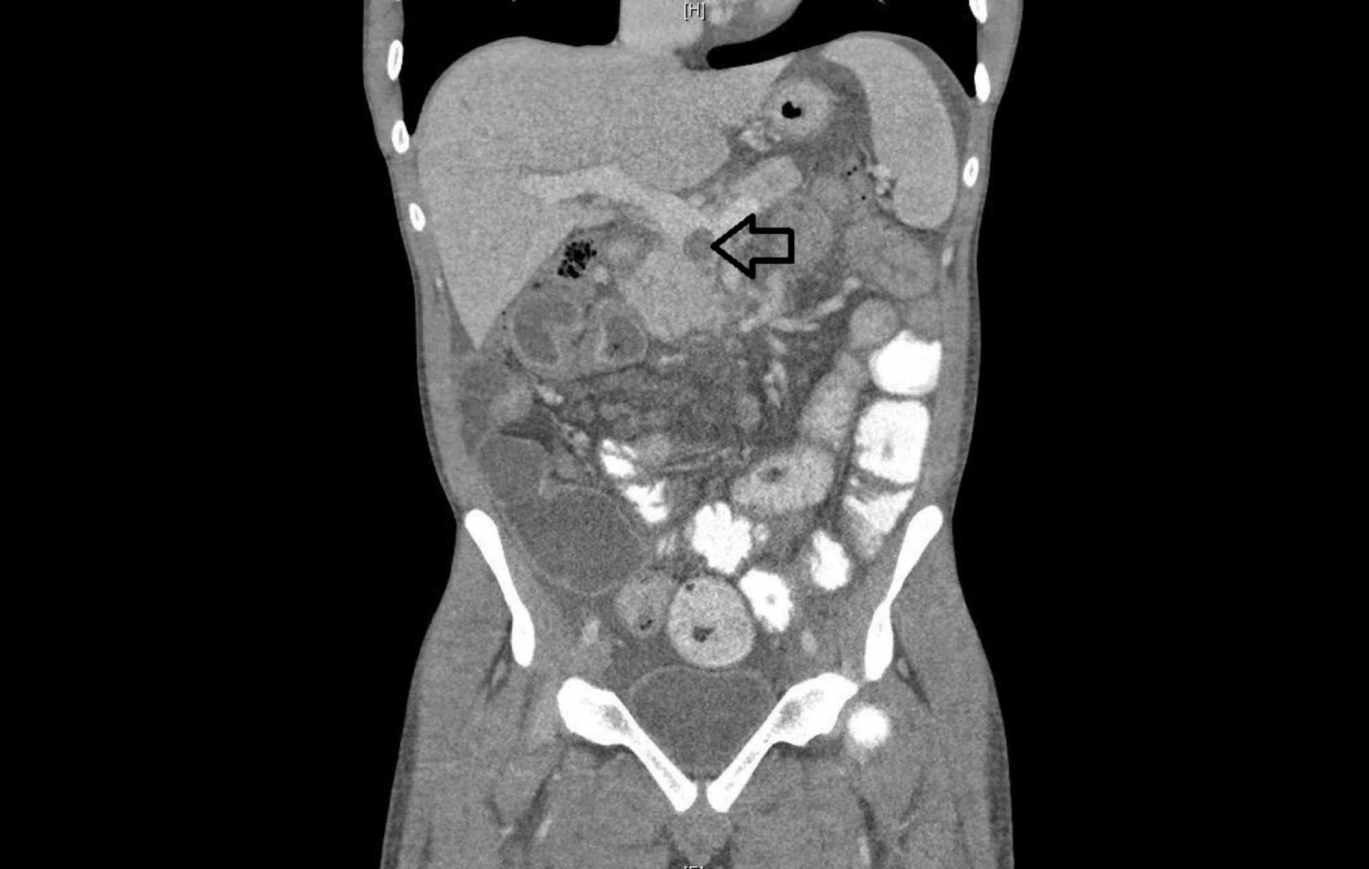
Sagittal view of the CT scan abdomen/pelvis showing mesenteric vein thrombosis (arrow) at the level of portal vein and splenic vein confluence.
